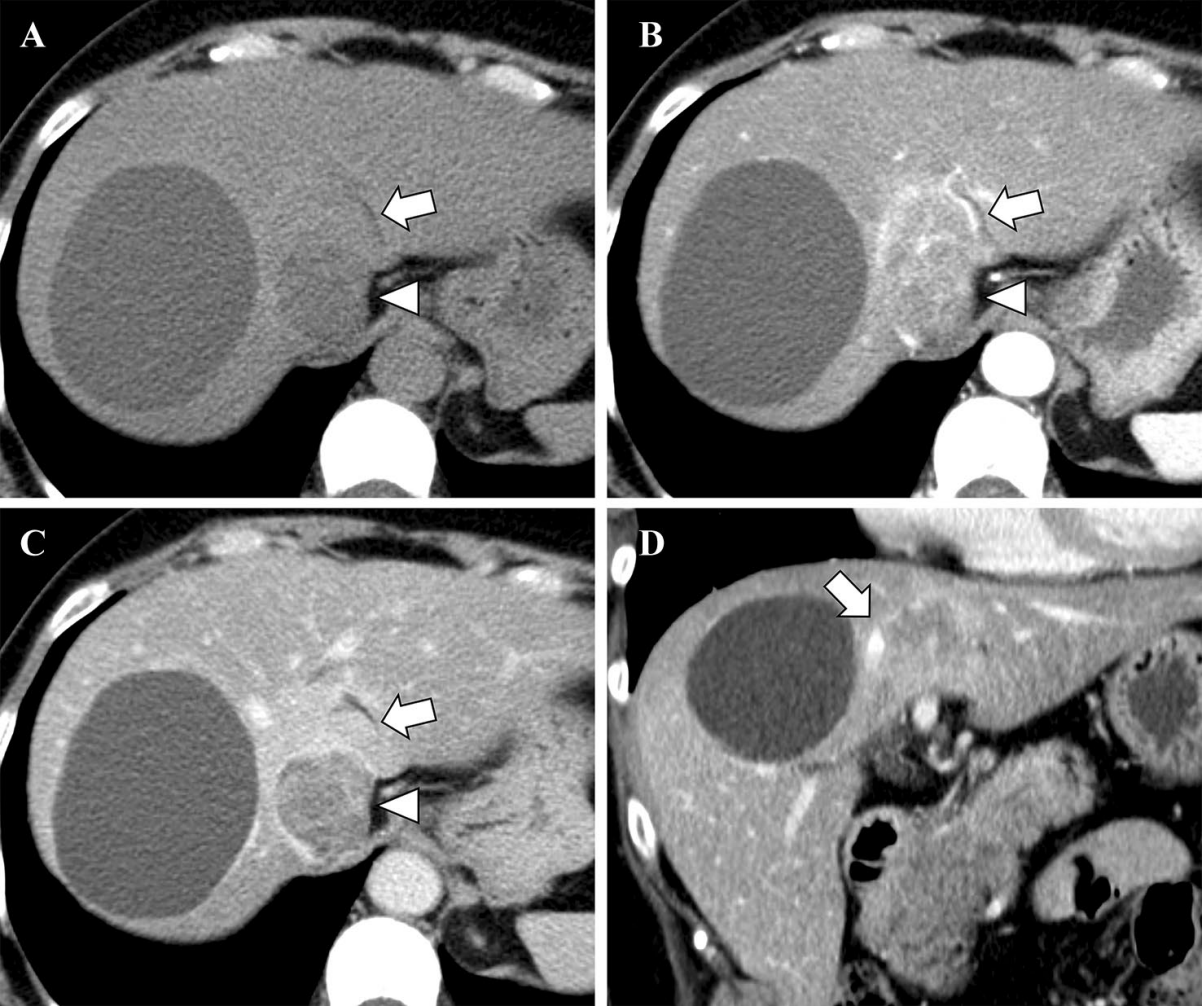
Abdominal CT demonstrates a tumor which is 50 mm in diameter in S1 of the liver. The ventral portion has an irregular margin and shows peripheral early and gradual centripetal enhancement (A plain CT; B arterial phase; C late/equilibrium phase, arrow). In the superior aspect of the lesion, the middle hepatic vein is obstructed, suggesting venous invasion of the tumor (D coronal plane in portal phase, arrow). The dorsal portion is a well-defined and low-attenuated mass and shows heterogeneous early enhancement and partial wash-out (A–C arrowheads)
